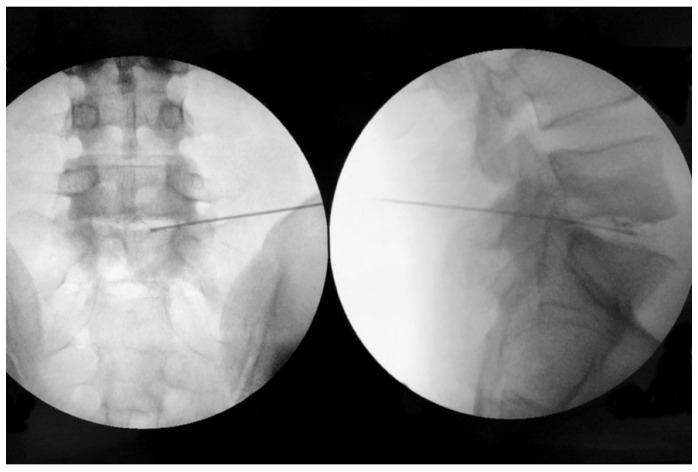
AP (left) and LAT (right) X-ray views of the ethanol gel injection under fluoroscopic guidance. The presence of tungsten in the gel provides a radiographic contrast effect as evidenced by contrast seen in the nucleus pulposus in the lateral view.
